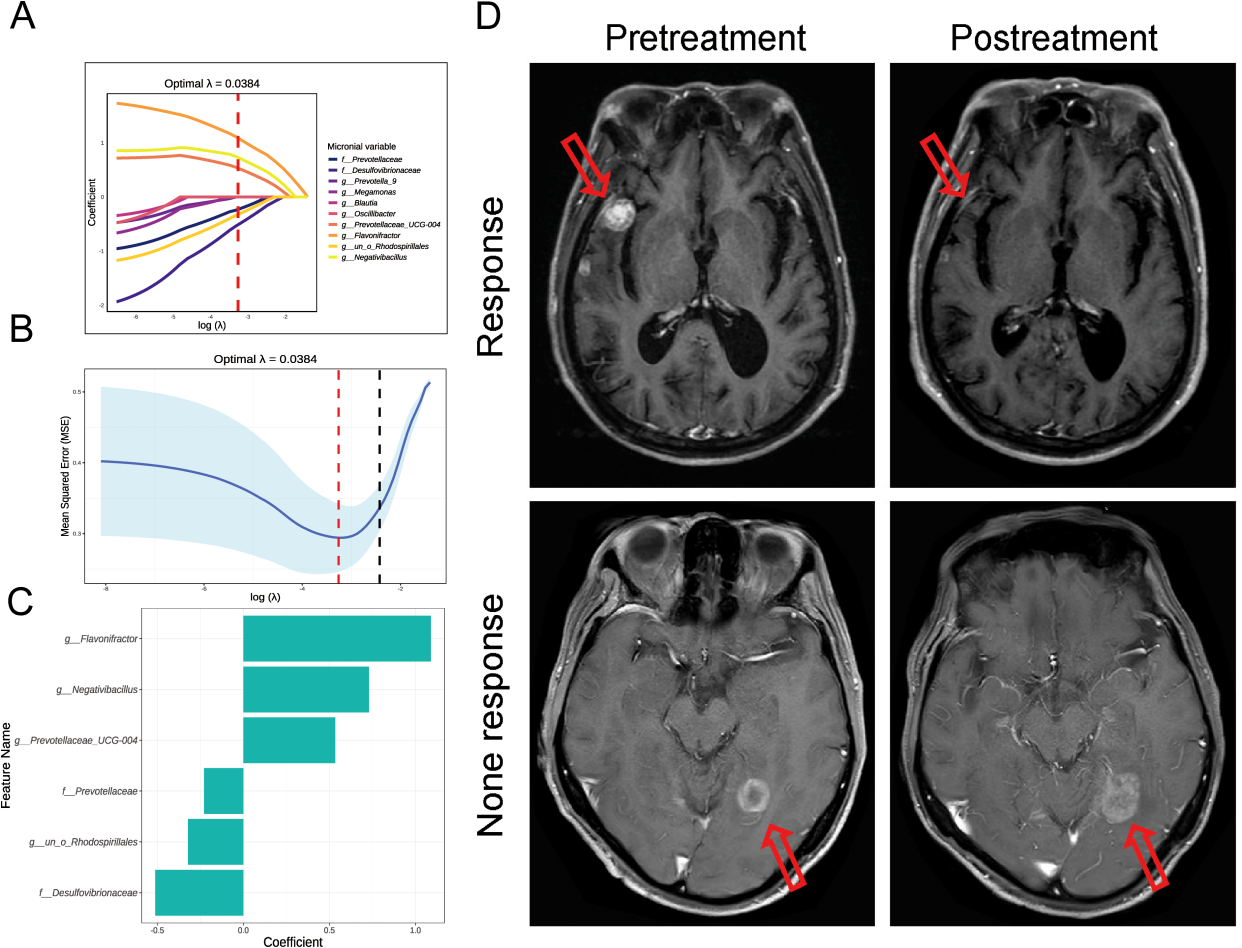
Construction of the LASSO model (microbial factors) and brain MRI scans of representative clinical cases. (A) Coefficient path plot for the 10 microbial features included. (B) Results of 10-fold cross-validation. (C) Six key microbial features selected by the LASSO model. (D) Upper panel: Patient 1 had a microbial score of 0.317, CRP of 10.75, and SIRI of 1.363. Using these values into the nomogram model formula, the linear predictor was -0.4306, which corresponds to a risk probability of 0.394 for no response. Post-treatment MRI showed near-complete resolution of the irradiated lesion, and the clinical outcome was consistent with the model’s prediction. Lower panel: Patient 2 had a microbial score of 1.339, CRP of 13.5, and SIRI of 5.005. Using the nomogram formula, the linear predictor was 2.0427, corresponding to a risk probability of 0.885 for no response. Follow-up MRI after radiotherapy indicated disease progression, and the clinical outcome matched the model’s prediction.
